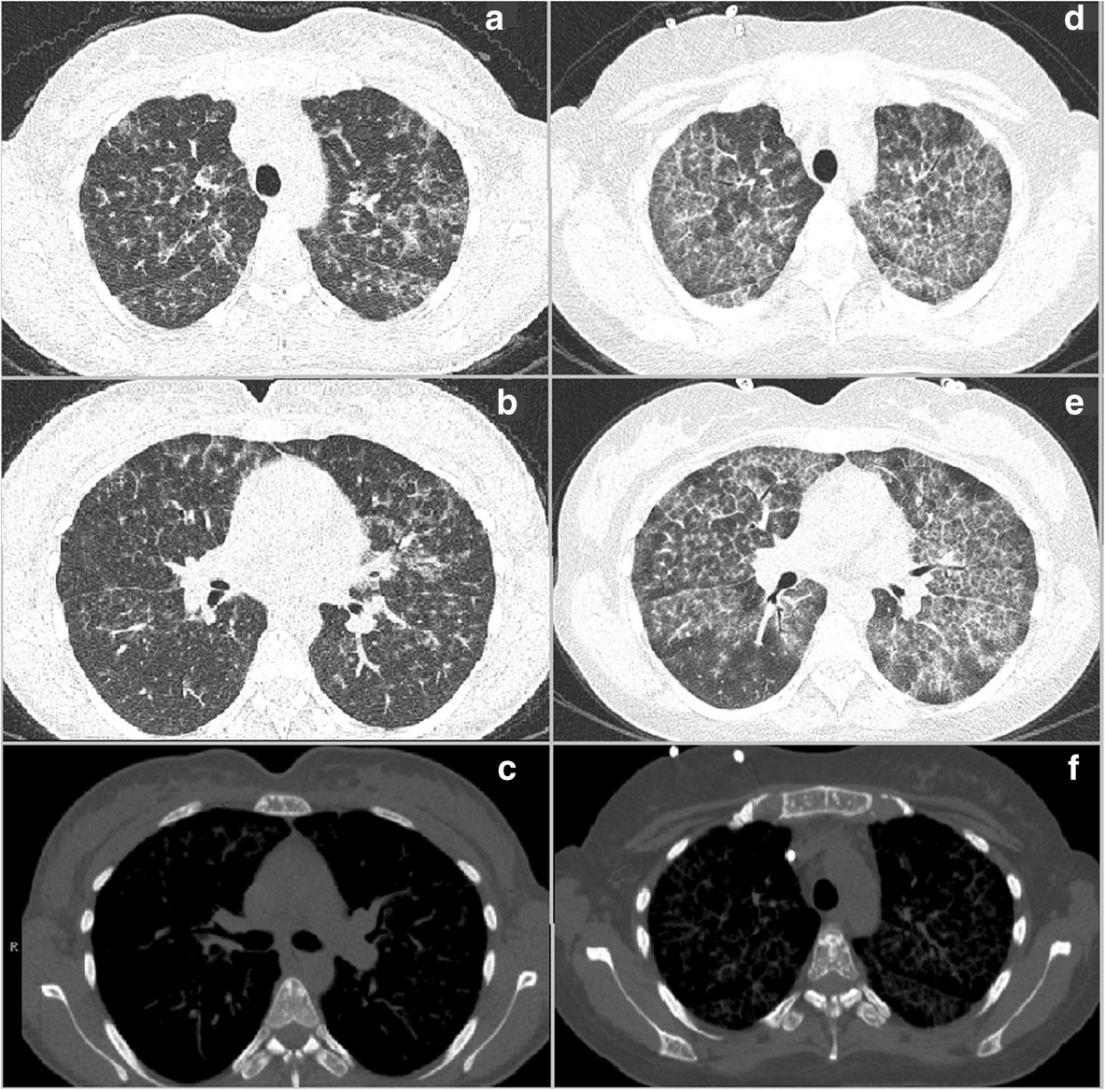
Computed tomographic images obtained at diagnosis (a-c) and after 4 months (d-f). a and b The first computed tomograms of the chest show a ground-glass aspect that is most pronounced in the apical areas with a diffuse reticular aspect and widening of interlobular septa. At this time, the groundglass was mainly localized around bronchovascular bundles. c and f The skeleton shows multiple focal sclerotic lesions. d and e Computed tomographic scans obtained 4 months after the first ones show progressive and diffuse reticular ground-glass lesions
